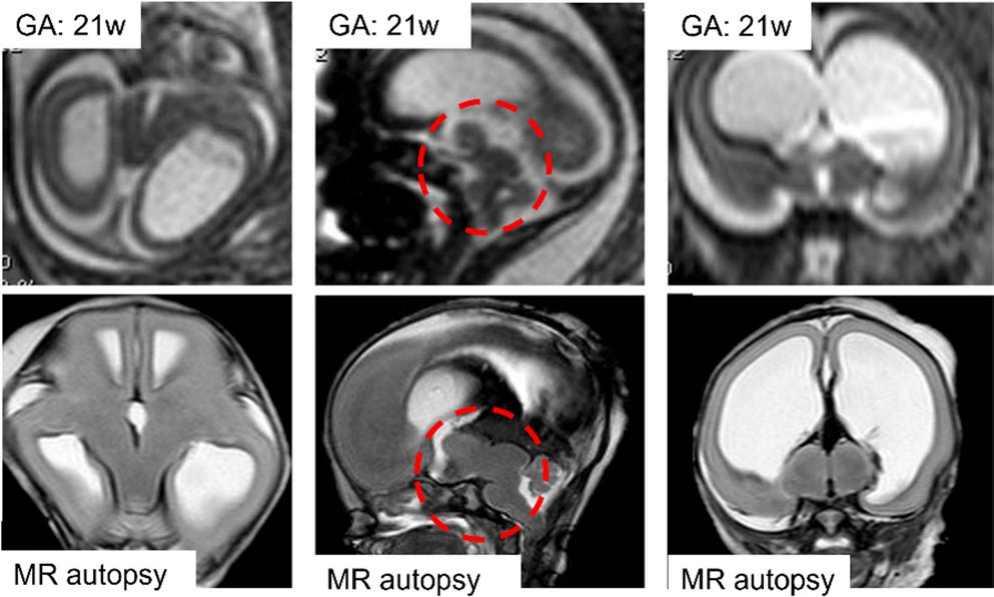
Axial, sagittal and coronal fast spin-echo T2w MR images of a 21-week fetus depict a poorly defined junction between the diencephalon and the mesencephalon, with a deep longitudinal cleft in the ventral midline of midbrain contiguous with the third ventricle, a caudal displacement of diencephalic-mesencephalic junction and an abnormal butterfly-shaped midbrain associated with hydrocephalus. These findings are confirmed on turbo spin-echo T2w MR-autopsy images
